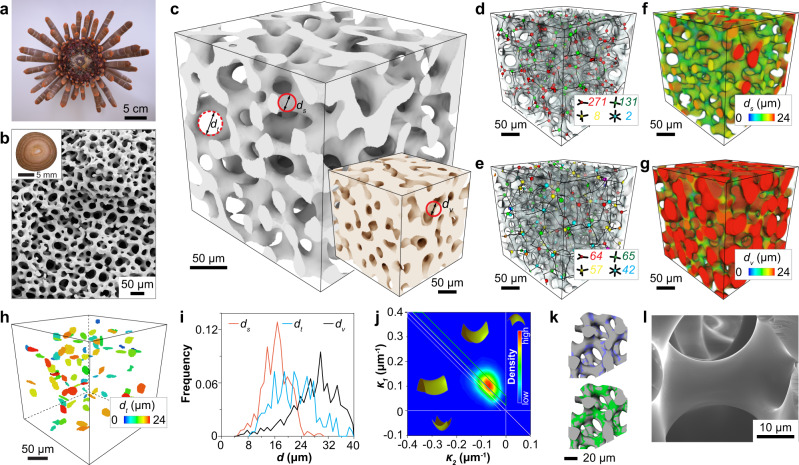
Echinoderm stereom as a bicontinuous cellular solid. a Photograph of a ventral-viewed sea urchin *H. mamillatus*. b SEM image of the stereom structure. Inset, optical image of the transverse cross section of a spine. c µ-CT reconstructions of stereom and the corresponding void structure (inset). *d*_*s*_, *d*_*v*_, and *d*_*t*_ represent the thicknesses (diameters) of stereom, void structure, and throats, respectively. d, e 3D cellular network of stereom and the corresponding void structure with node types colored by their connectivities. f, g The thickness distributions of stereom (*d*_*s*_) and the corresponding void structure (*d*_*v*_). h 3D rendering of small throats (*d*_*t*_ < 24 μm) for volume (c). i Distribution of *d*_*s*_, *d*_*v*_, and *d*_*t*_. j Interfacial shape distribution of stereom. *κ*_1_ and *κ*_2_ are the maximum and minimum principal curvatures, respectively. k Visualization of regions in stereom with curvature distributions shown in (j), where purple and green regions correspond to minimal surfaces with zero mean curvature and the saddle surface with the highest distribution density, respectively. l An SEM image of the branch surface of stereom.
